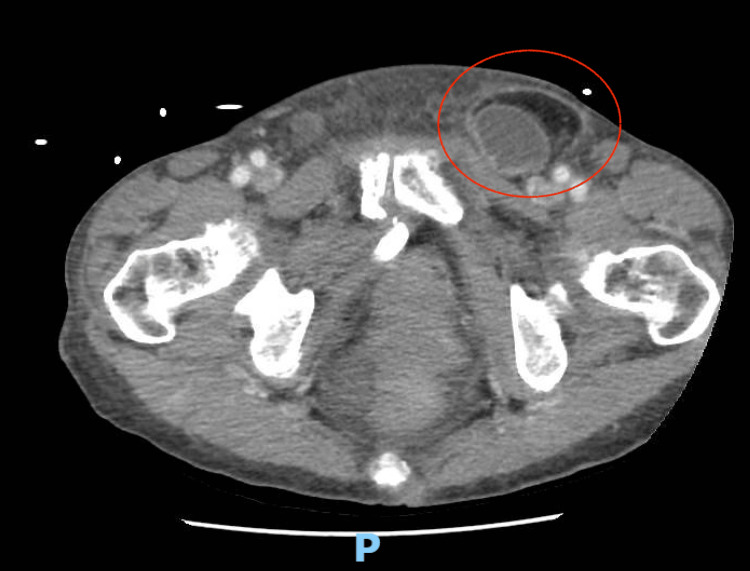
CT image depicting left lower abdominal mass containing bowel.
